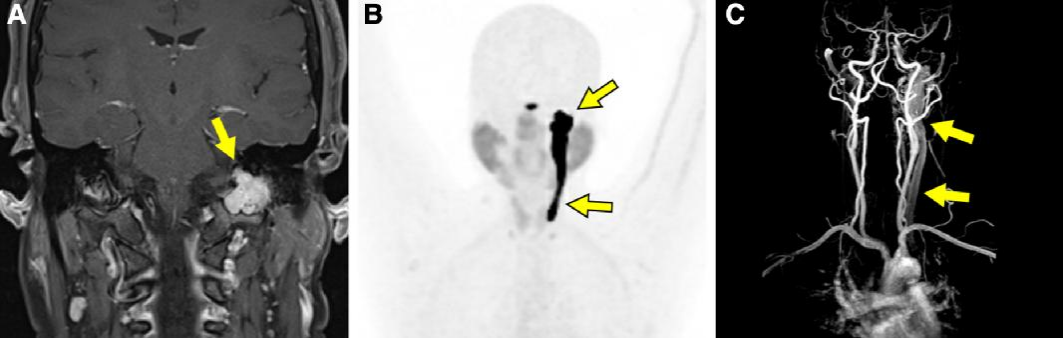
Magnetic resonance imaging, ^64^Cu-DOTATATE positron emission tomography and magnetic resonance angiography of the skull base and neck.
